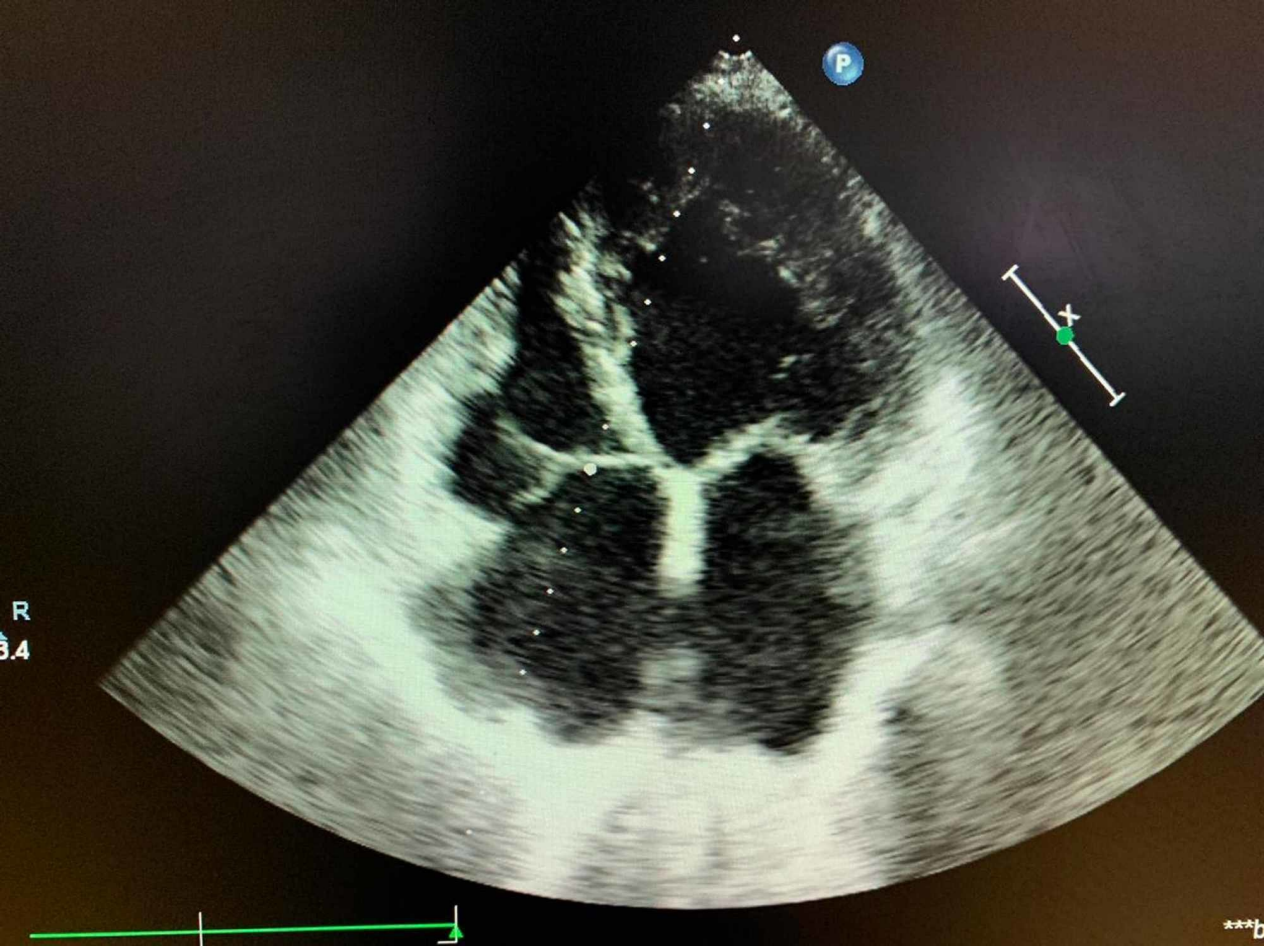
Echocardiography shows global hypokinesia, with preserved wall thickness
